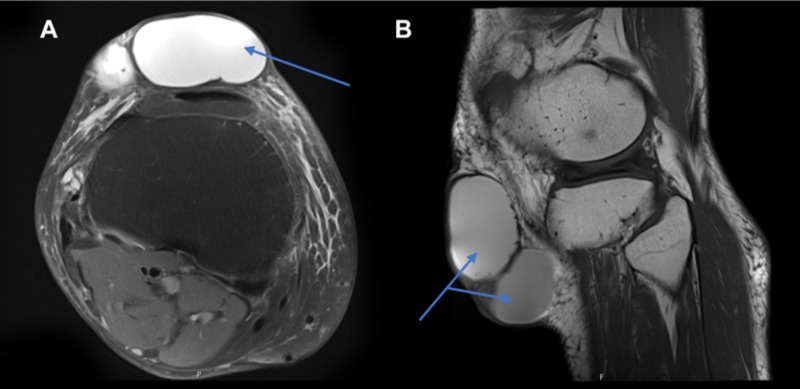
Magnetic resonance imaging (MRI) of the right knee with contrast showing loculated cystic mass A. Axial MRI of the right knee with gadolinium contrast showing a subcutaneous mass (see arrow) on the anterior aspect of the tibia and knee joint. It consists of a large loculated mass containing some debris inferiorly, lying superficial to and separate from the infrapatellar tendon. The mass has high signal on T1 with no enhancement around the cyst. B. Sagittal MRI of the right knee showing a subcutaneous mass (see arrows).
